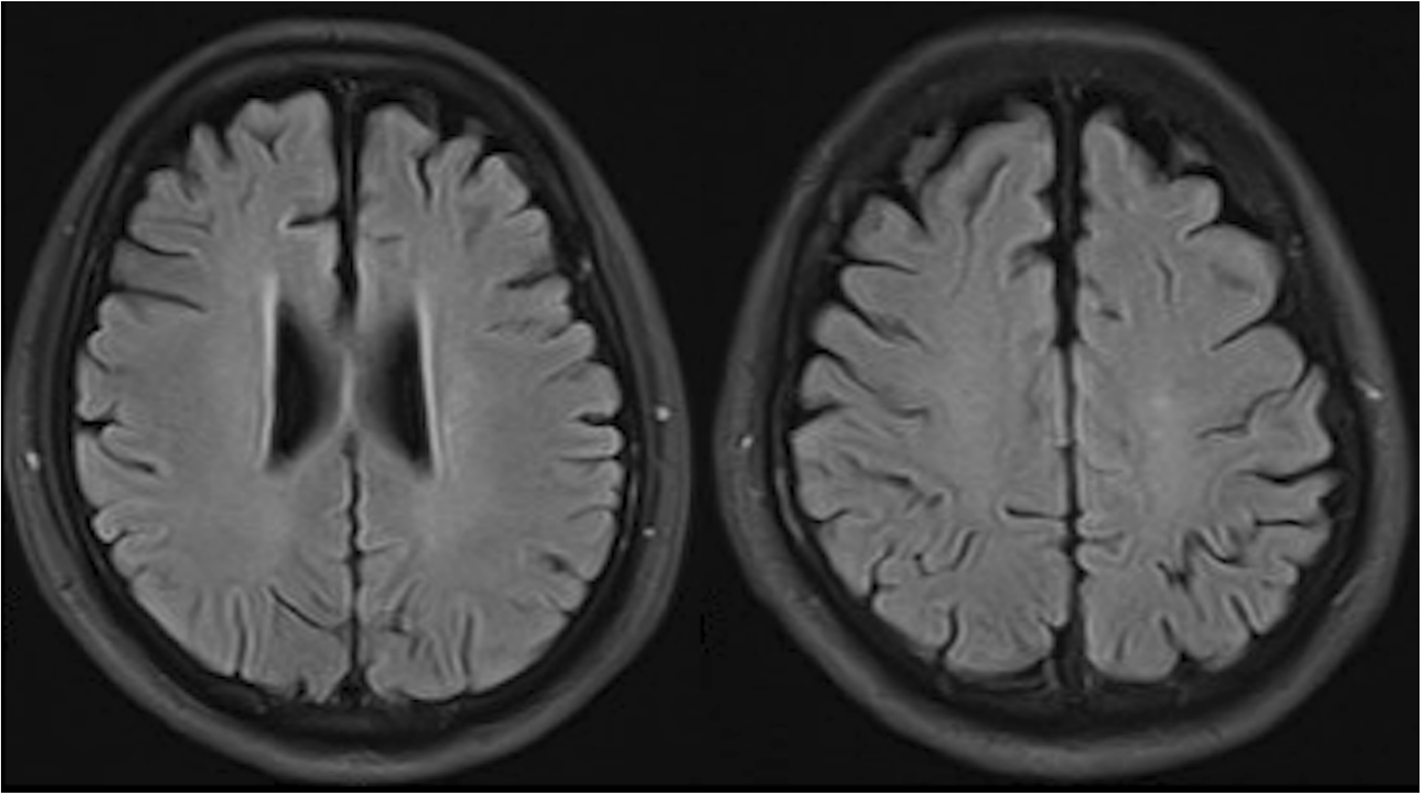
Head MRI of this patient re-examined 11 months after the car accident showed that the “star field” pattern lesions were almost completely absorbed
